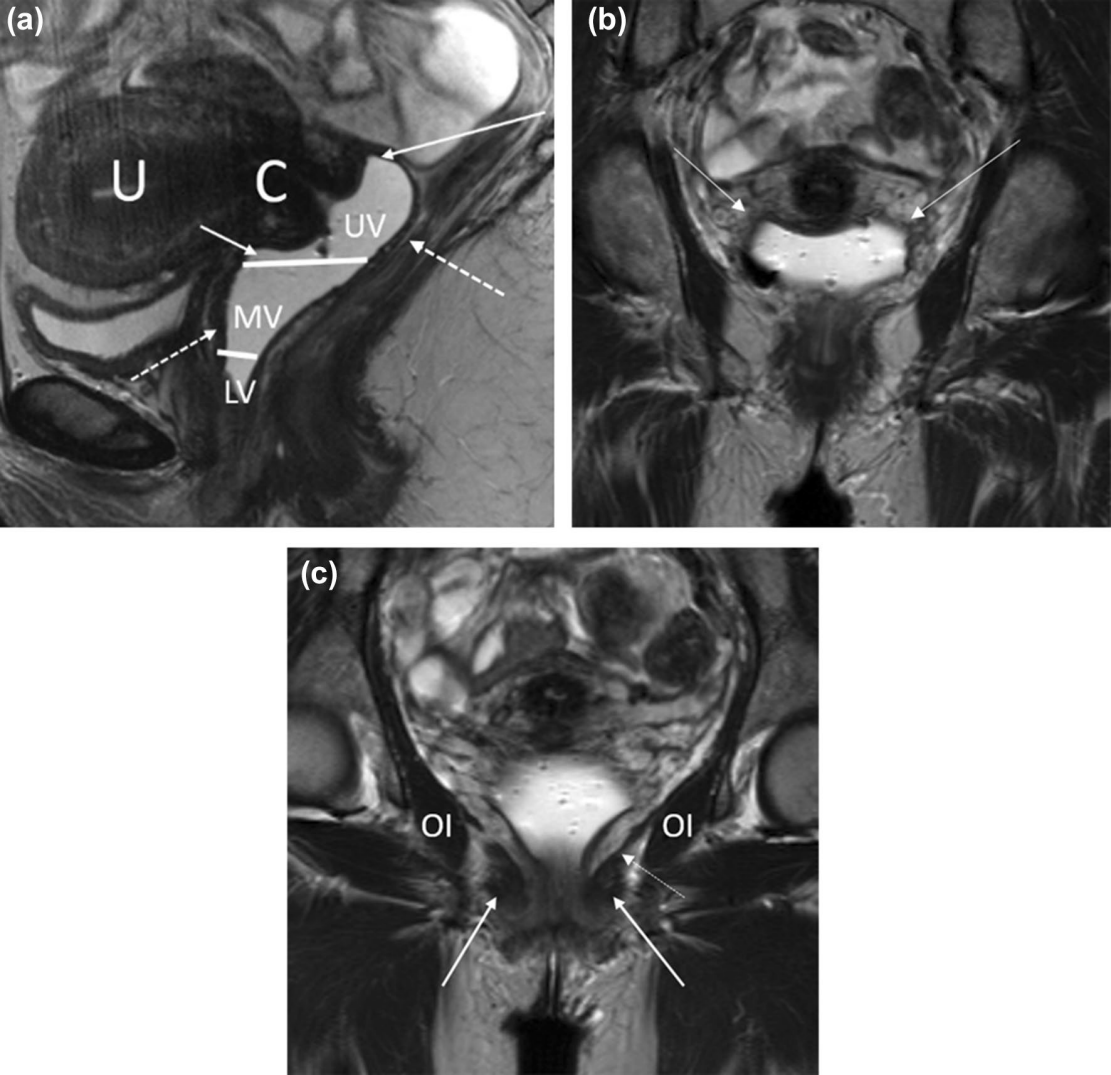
Relevant MR anatomy of the vagina. a Sagittal T2-weighted MR image of the vagina (distended with gel) shows the upper (UV), middle (MV), and lower (LV) thirds. The image also shows the anterior and posterior fornices (solid arrows), cervix (C), and uterus (U). The dotted arrows demonstrate the T2-hypointense muscularis layer of the vagina. b Coronal T2-weighted MR image of the vagina shows the lateral fornices of the vagina (solid arrows). c Coronal T2-weighted MR image through the vagina demonstrates the puborectalis (solid arrow), levator ani (dotted arrow), and obturator internus (OI)
